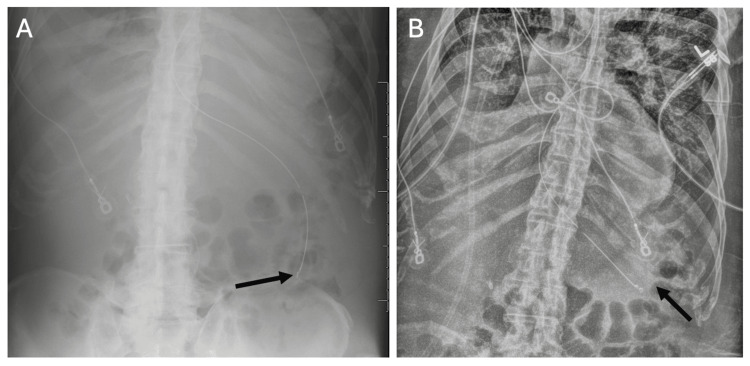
Correct Positioning of a Nasogastric (NG) Tube on Radiographs Radiographs demonstrating appropriate placement of a nasogastric tube. (A) NG tube coursing midline through the esophagus, crossing the diaphragm, and terminating within the gastric body (arrow). (B) Distal tip projecting over the stomach, below the left hemidiaphragm (arrow). Proper recognition of these features is essential to distinguish correct intragastric positioning from esophageal or airway malposition.
